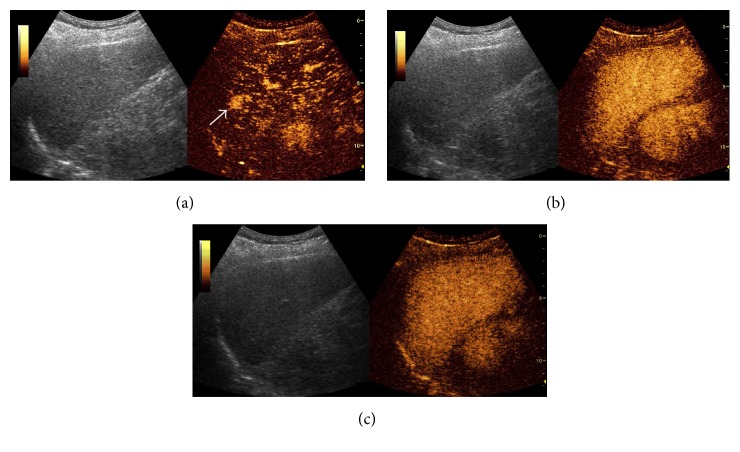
Small recurrent lesion (15 mm) indistinctive on B mode ultrasound showed homogeneous hyperenhancement during arterial phase (a). Isoenhancement during the portal venous (b) and late phase (c) on contrast-enhanced ultrasound. The arrow in (a) refers to homogeneously hyperenhanced small recurrence lesion during arterial phase.
